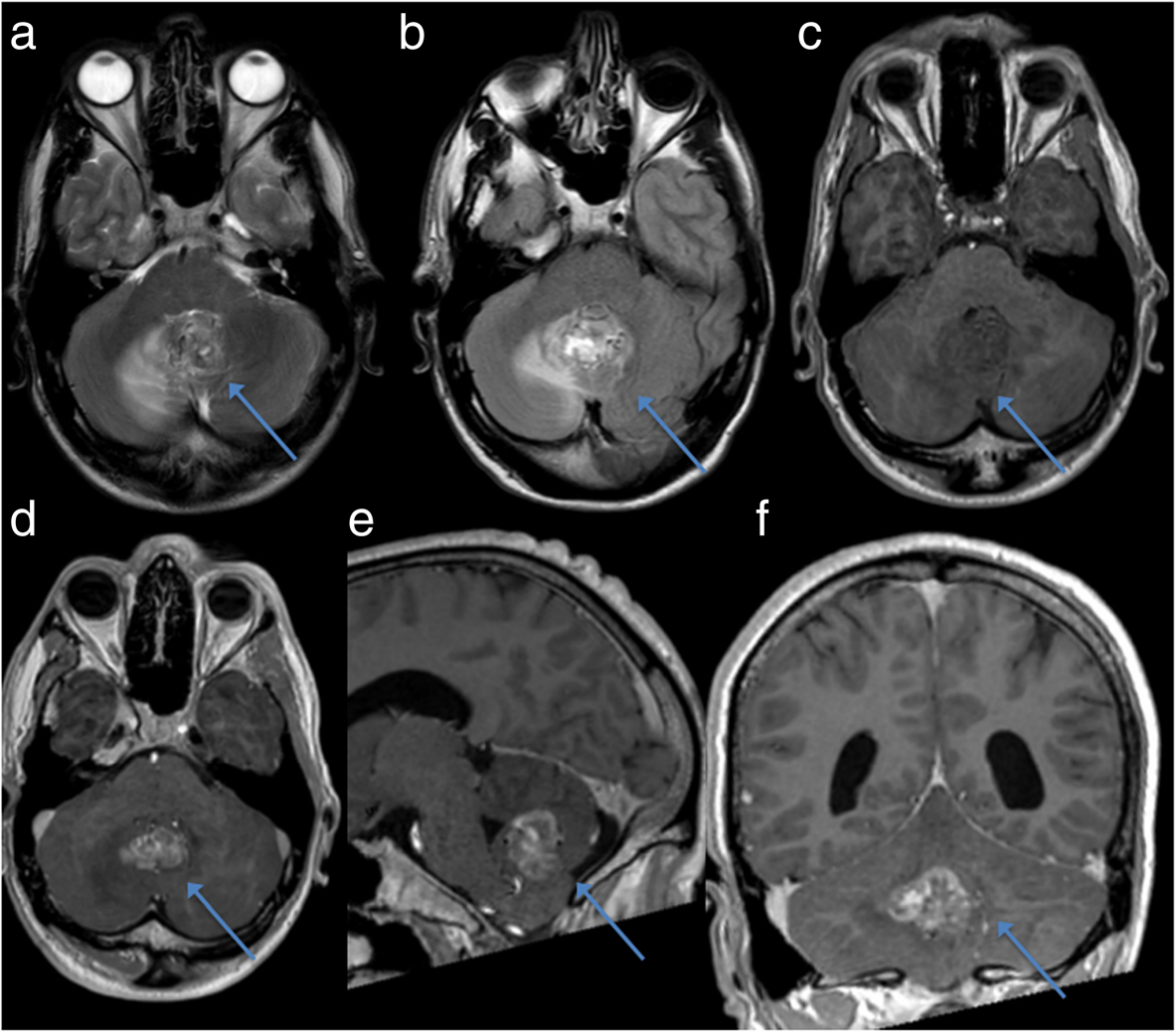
Pre-operative MRI brain demonstrating a heterogeneous, enhancing posterior fossa lesion with surrounding edema (blue arrow). 1a: Axial T2-weighted; 1b: Axial FLAIR; 1c: Axial T1-weighted pre-contrast; 1d: Axial T1-weighted post-contrast; 1e: Sagittal T1-weighted post-contrast; 1f: Coronal T1-weighted post-contrast
